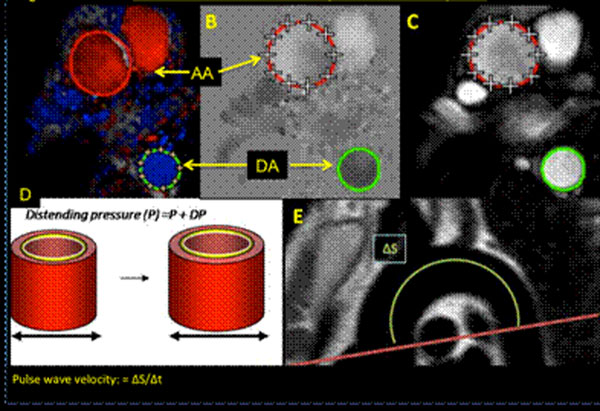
Aortic stiffness and distensibility measurements by MRI
